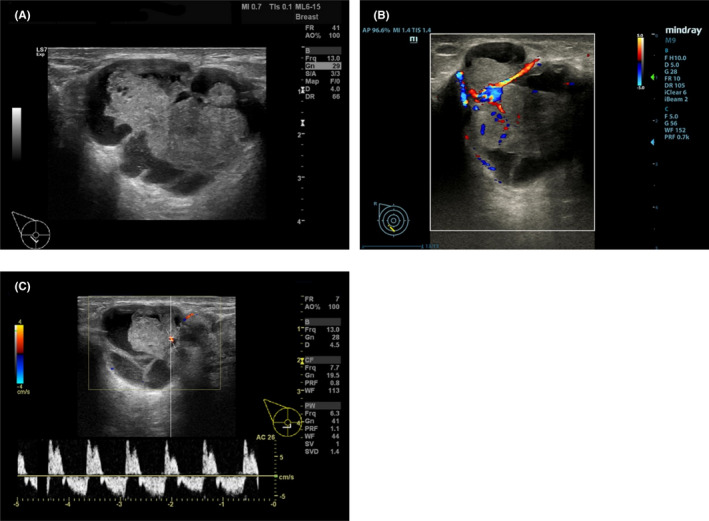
Ultrasonography of primary breast mucoepidermoid carcinoma. (A) Gray‐scale ultrasound showed the mass was irregular, lobulated, and well‐defined. (B) Color Doppler ultrasound showed the solid tissue with rich blood flow signals. (C) Pulsed‐wave Doppler ultrasound showed the blood flow had high resistance
